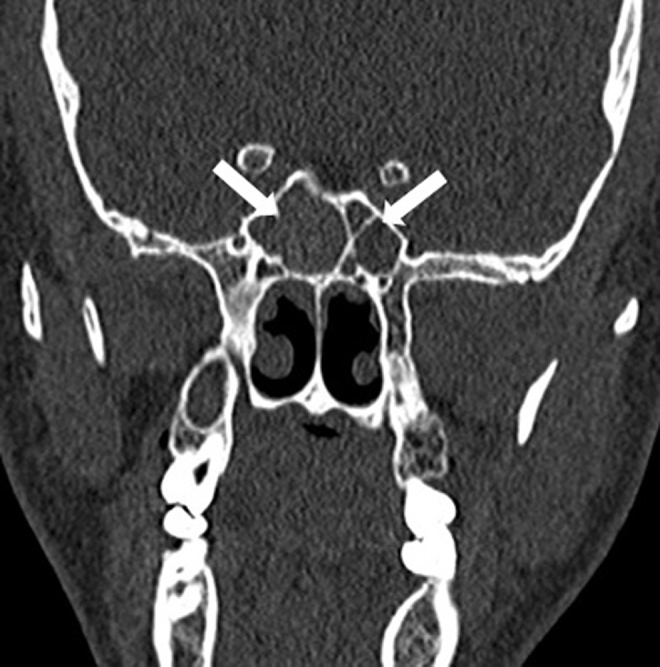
The CT scans of the paranasal sinuses shows bilateral sphenoiditis (arrows).
